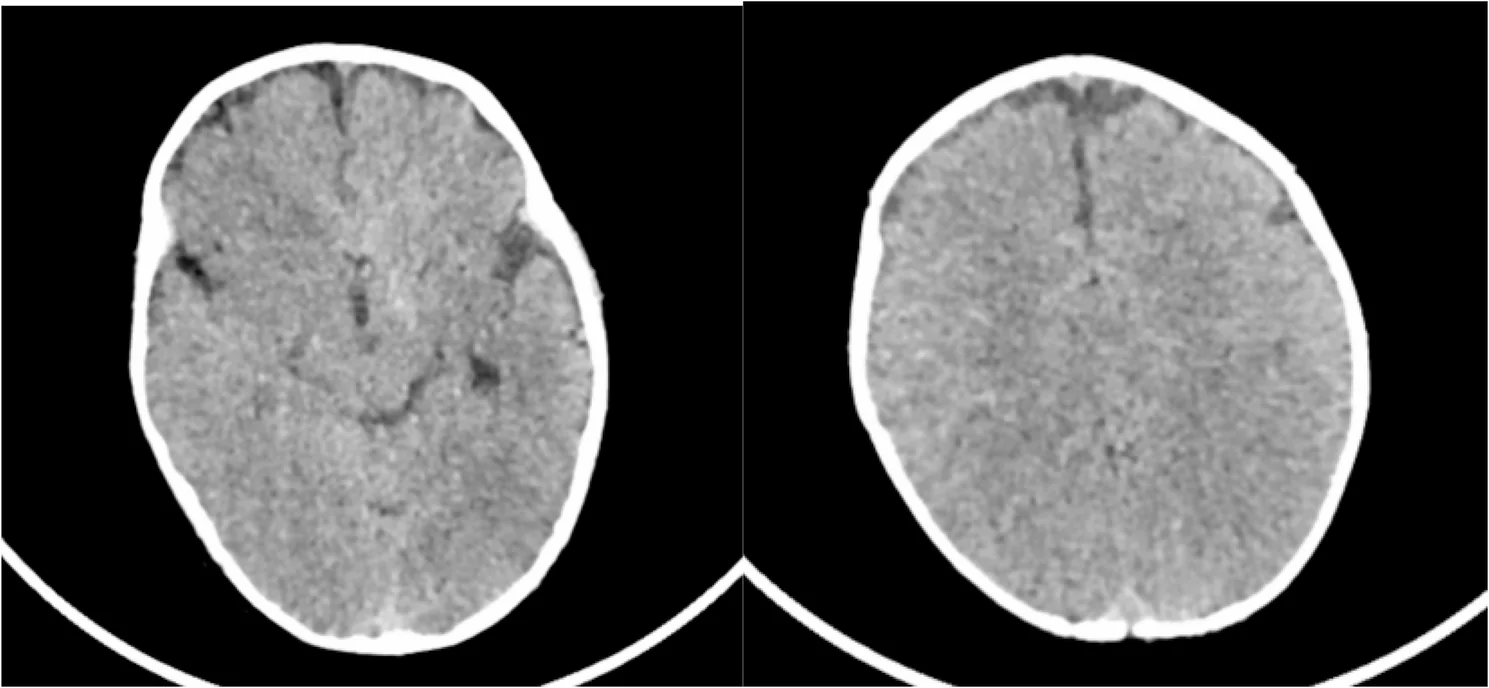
Axial brain CT scan revealing no parenchymal lesions or extra-axial collections
